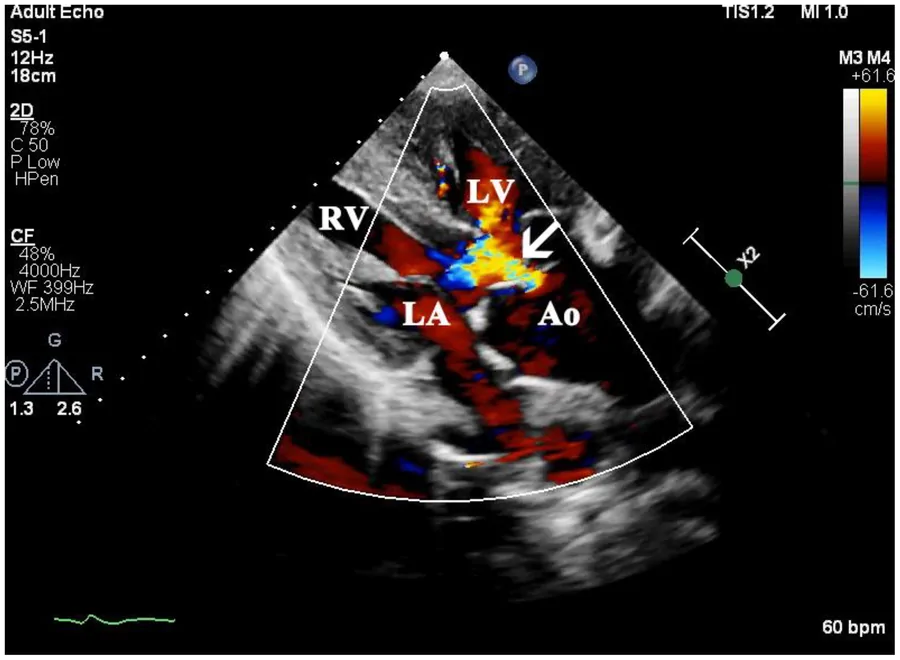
Preoperative transesophageal echocardiography showing dilated aortic root with color Doppler evidence of aortic regurgitation.
